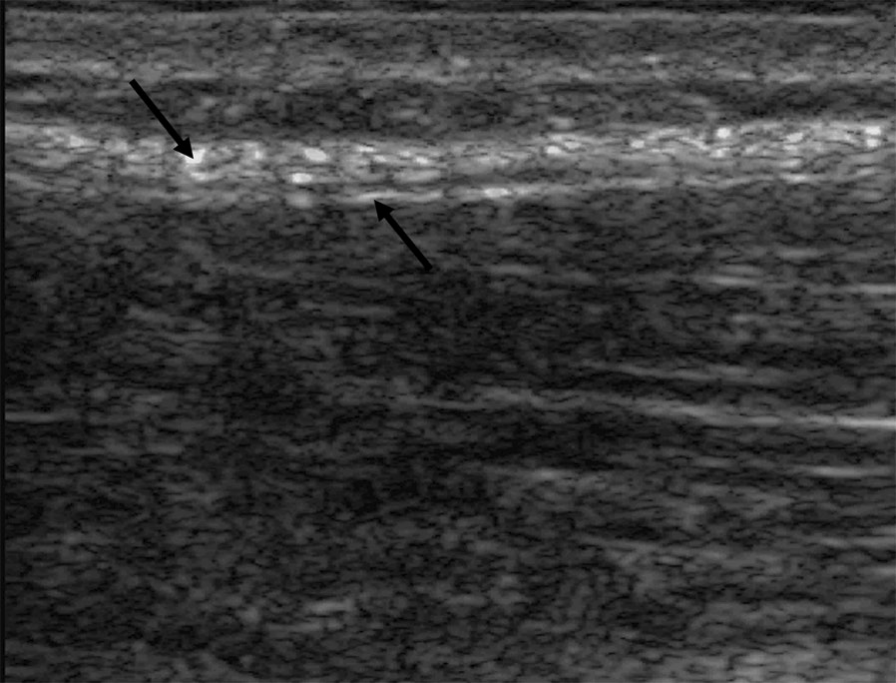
Parasagittal ultrasound image of thoracolumbar fascia at the L2-3 level. Left downward arrow: Thick echogenic layer representing the aponeurosis of the latissimus dorsi and abdominal muscles. Right upward arrow: Thin echogenic layer representing the aponeurosis surrounding paraspinal muscles. Between the arrows is a thin echolucent layer of loose connective tissue. Deep to the thin echogenic layer are paraspinal muscles. Shear strain represents differential motion (moving laterally on the image) between echogenic layers
